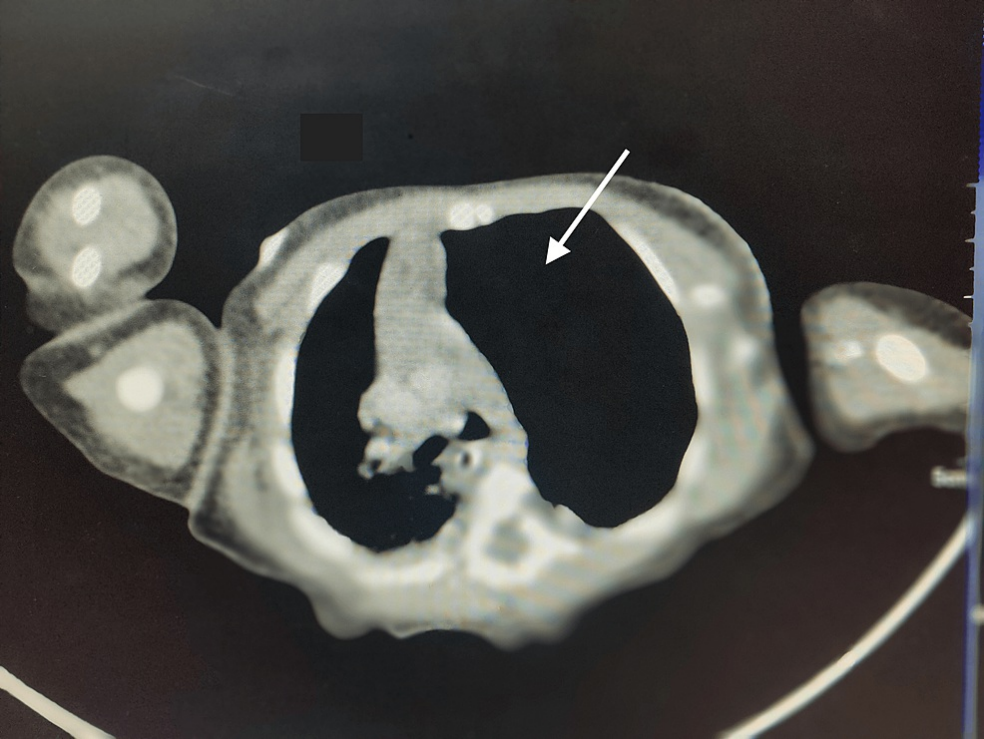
CT scan showing hyperinflation of the affected lobe with mediastinal shift (white arrow). CT: computed tomography.
